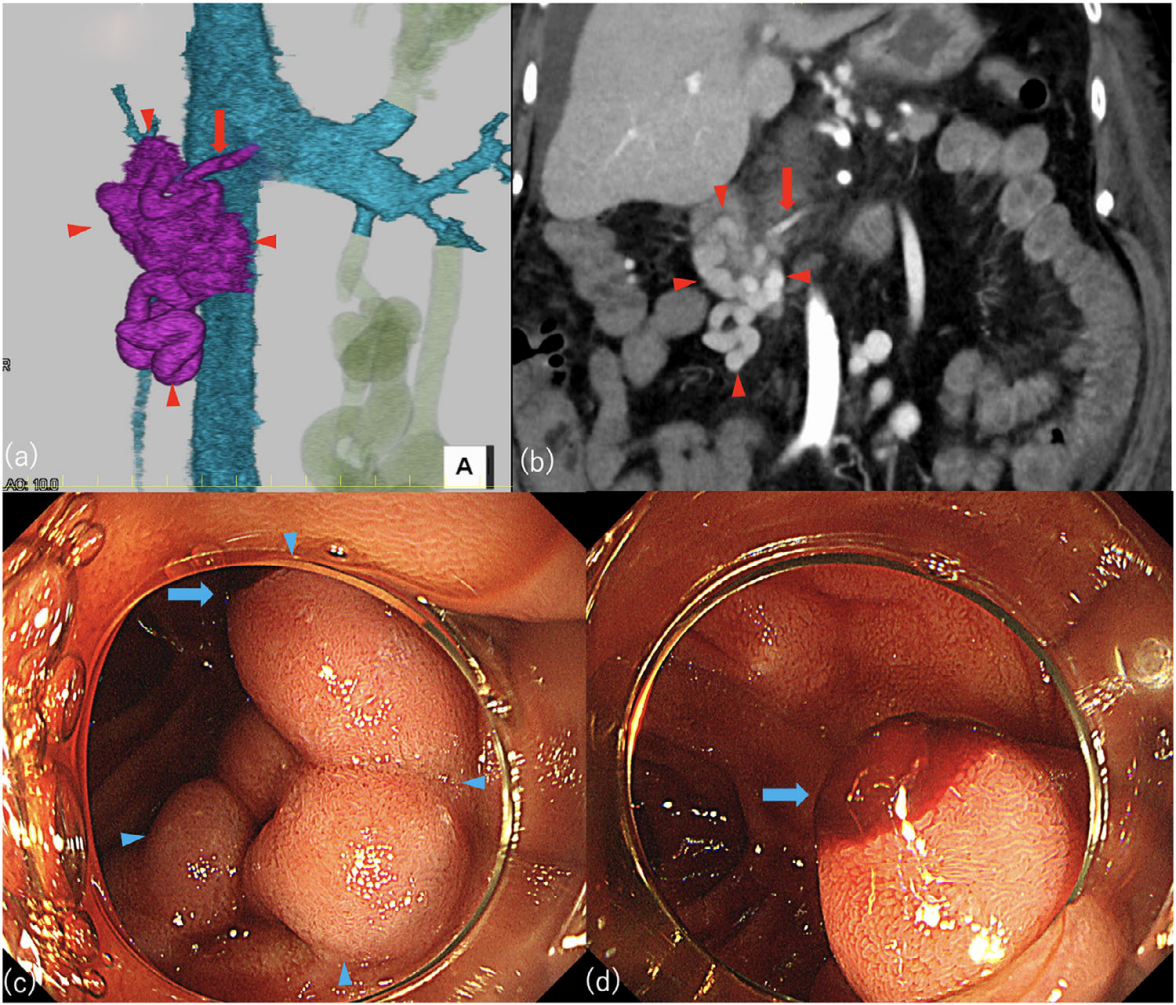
Contrast‐enhanced computed tomography images of duodenal varices and pre‐treatment endoscopic images of duodenal varices. (a) Three‐dimensional reconstructed image. (b) Coronal section. (c) Endoscopic view showing all the duodenal varices. (d) Endoscopic close‐up of the rupture site. Red arrowhead: duodenal varices. Red arrow: feeder vein originating from the proximal superior mesenteric vein. Blue arrowhead: duodenal varices. Blue arrow: rupture site.
